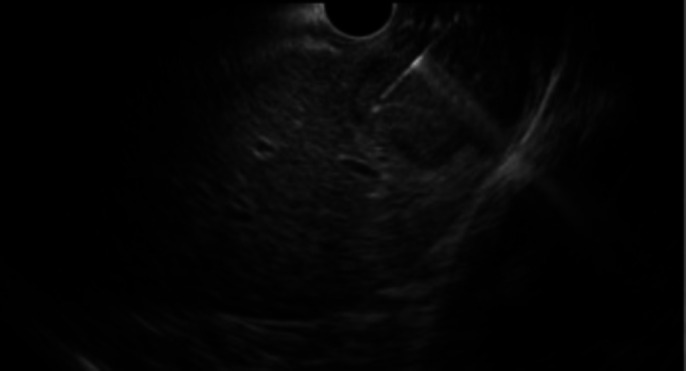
Endoscopic ultrasound showing metastatic liver lesions.
